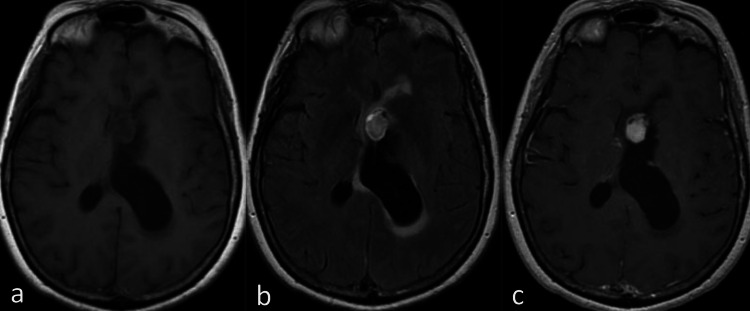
MRI findings Axial T1 w/o (A), T2 Flair (B), and T1 with (C) MR images. The lesion arises at the level of the foramen of Monro and is T1 hypointense and T2 hyperintense. Periventricular T2 hyperintensity and T1 hypointensity indicate transependymal edema, sequelae of ventricular dilatation, and elevated intraventricular pressure. There is heterogeneous enhancement of the lesion.
